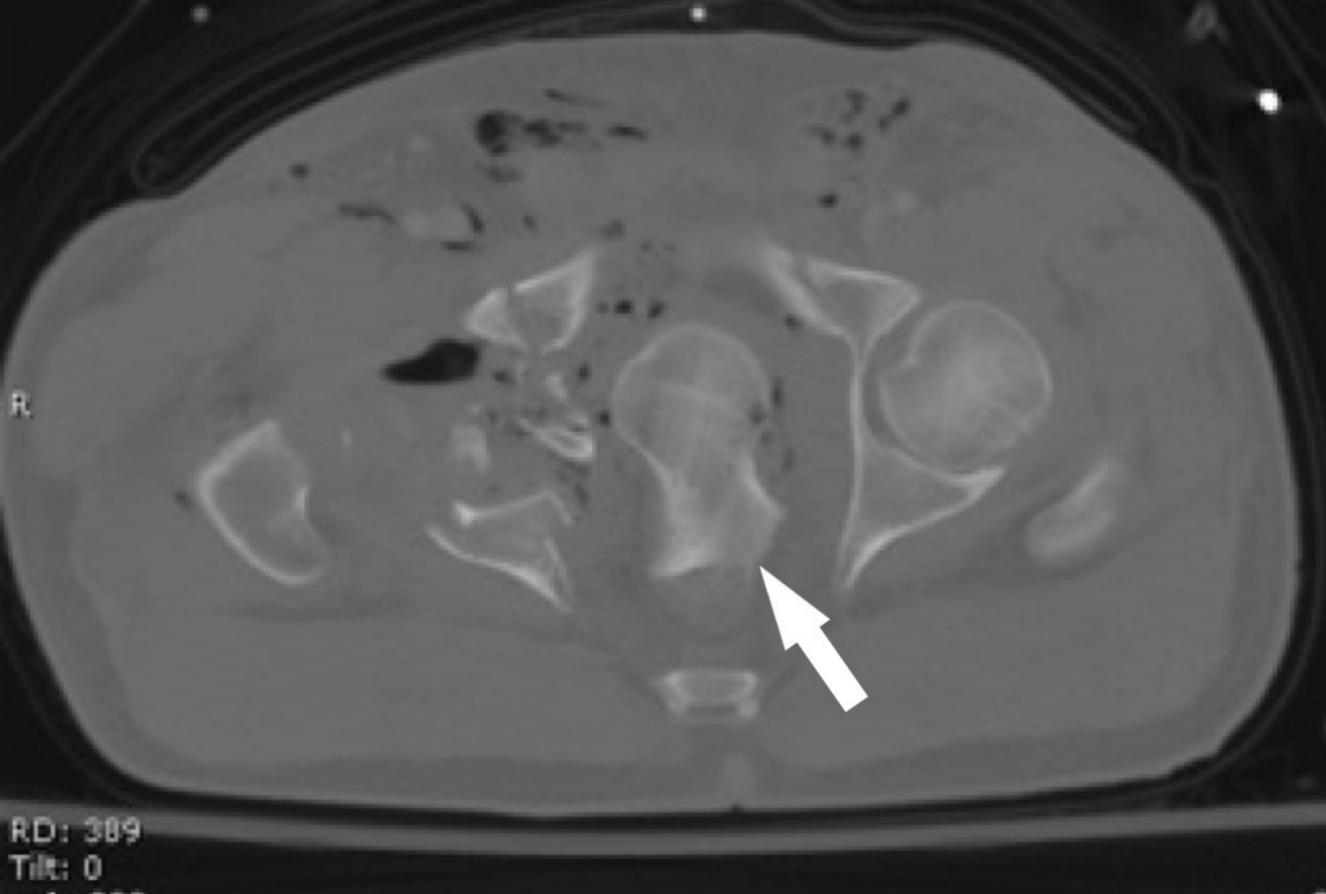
Axial plane CT imaging in the bone window setting showing the right femoral head (white arrow) penetrating the right acetabulum and right lateral wall of the rectum. CT: Computed tomography.
